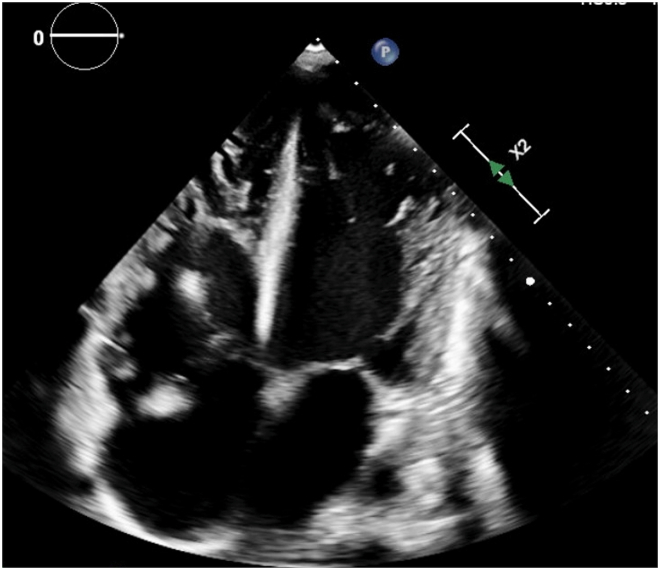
Initial Transthoracic Echocardiogram: Apical 4-Chamber View
